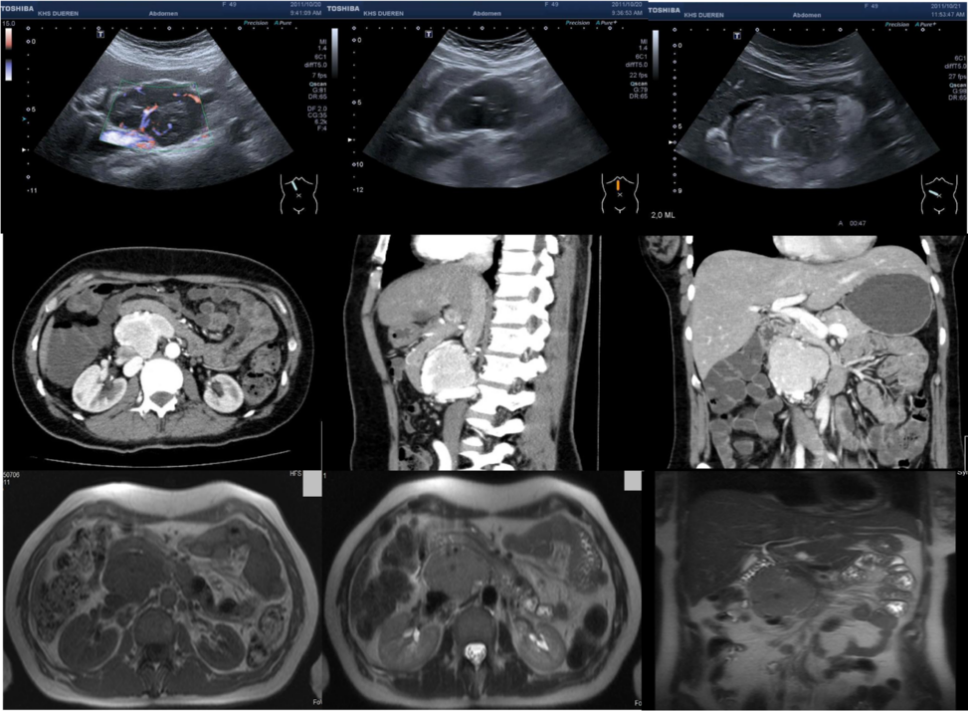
Pre-operative ultrasound, computed tomography and magnetic resonance imaging scans showing an interaortocaval tumor mass of approximately 6.3×4.7×6.8cm (measured on computed tomography scan).
